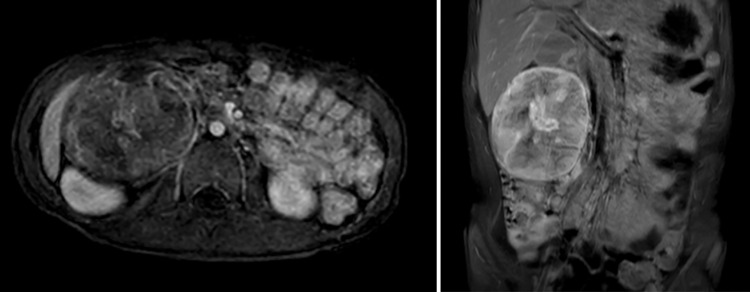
Abdominal MRI with contrast. A solid mass 78 × 61 mm in diameter was identified in the right lower renal region on MRI. The contrast-enhanced MRI scan shows heterogeneous enhancement of the lesion, especially in the central part of the tumor. MRI, magnetic resonance imaging
